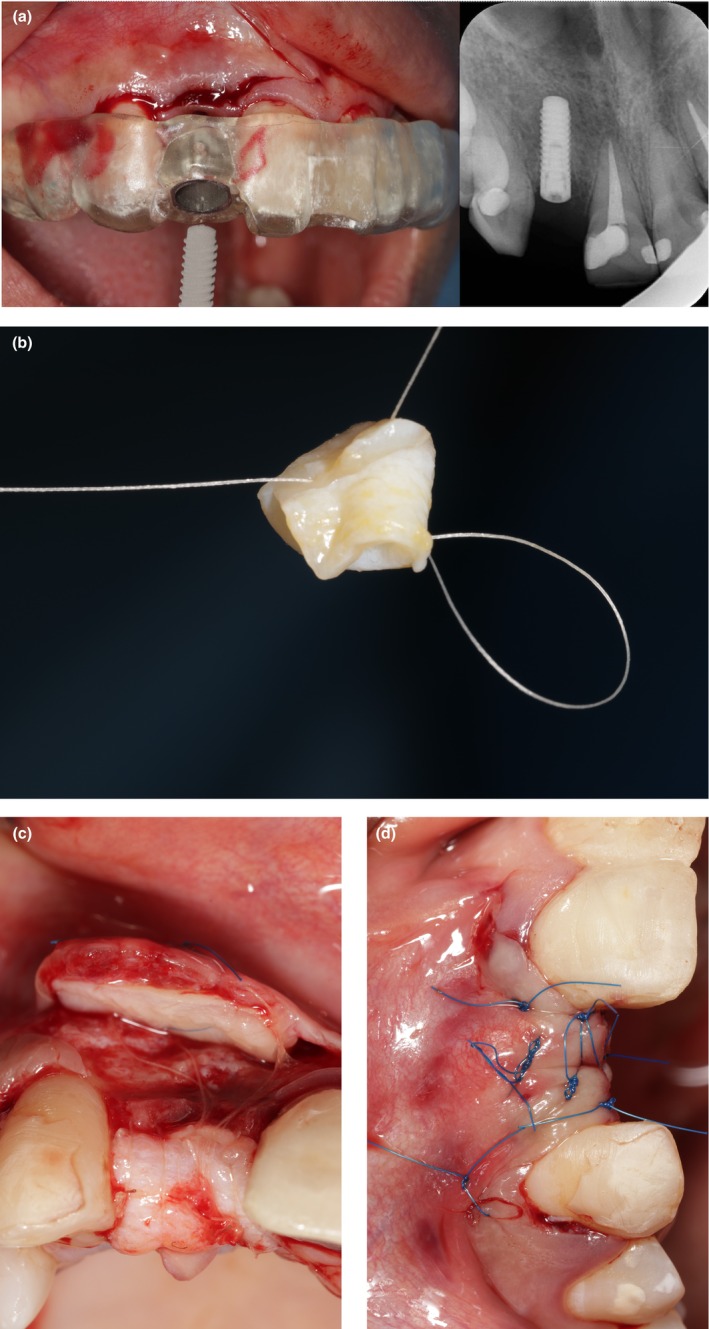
(a) Implant Insertion. A Straumann implant 3.3 × 10 mm was inserted using a digitally designed guide, which is depicted in periapical radiography. (b) Crestal de‐epithelized FGG. A rolled de‐epithelized FGG was attached using a PGA 6.0 suture to enhance the papilla height at the crest. (c) Buccal de‐epithelized FGG. The second part of the de‐epithelialized FGG was securely fixed using PGA 6.0 through single interrupted suturing to the underlying periosteum in the buccal area. (d) Flap closure. The flap was closed with nylon 6.0 and interrupted sutures.
